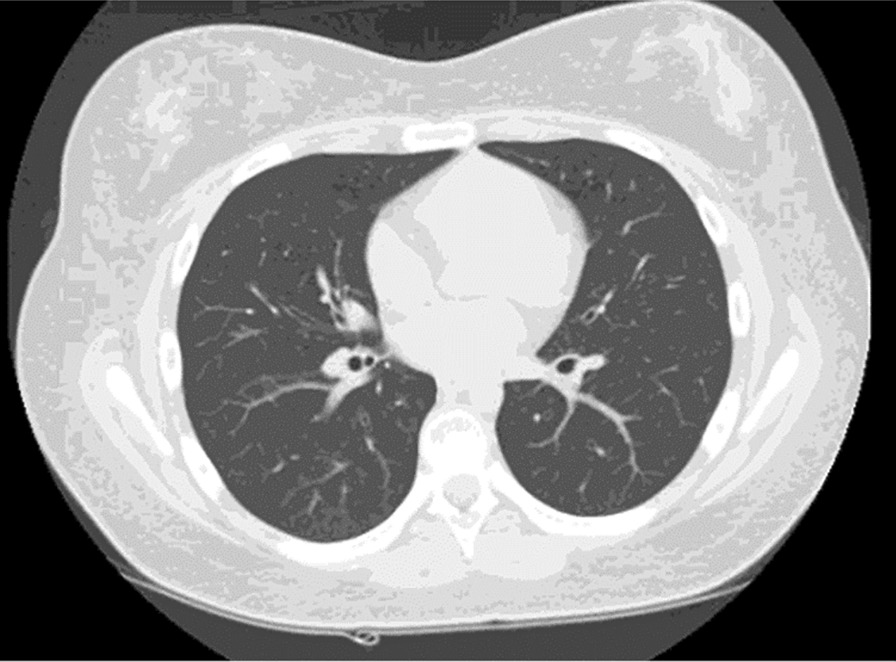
Chest computed tomography (CT) scan. The new chest CT scan shows the disappearance of pneumomediastinum and regular pulmonary expansion
